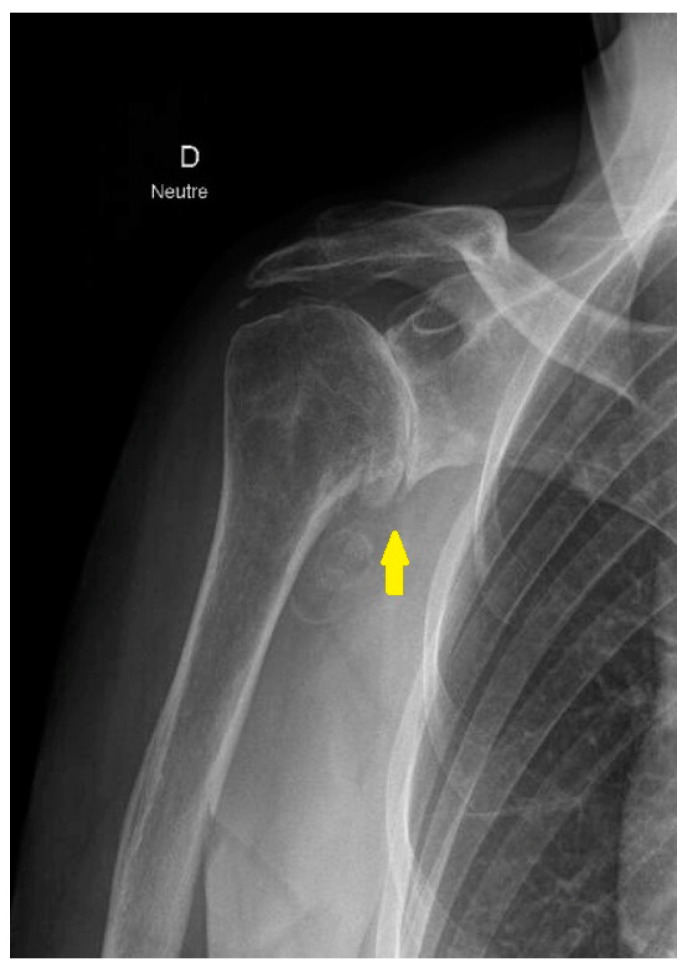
Radiograph of the anteroposterior projection of the shoulder with primary glenohumeral osteoarthritis. The yellow arrow highlights complete joint space loss, secondary to cartilage degeneration, subchondral sclerosis, and osteophyte formation—hallmarks of concentric osteoarthritis.
